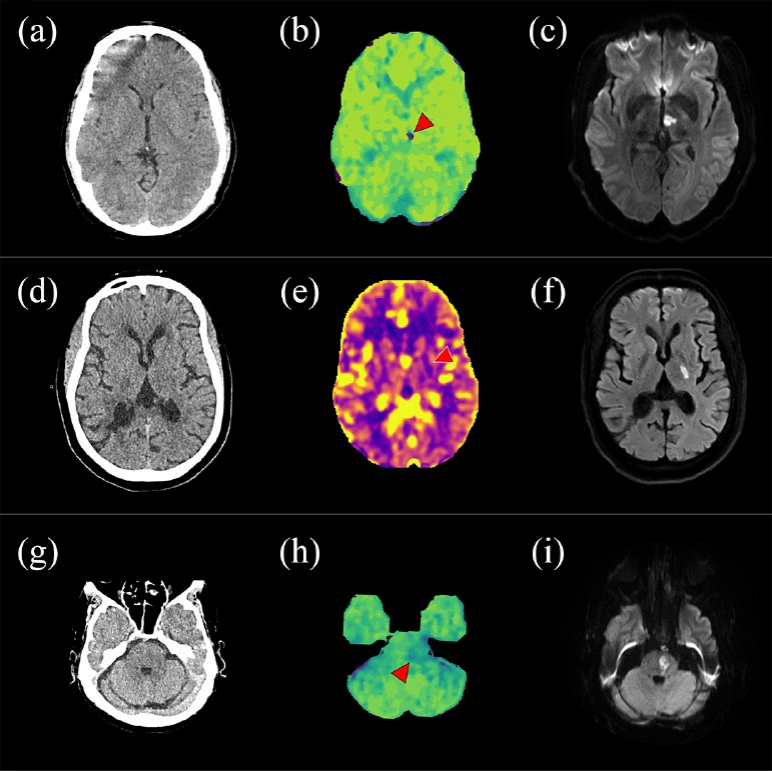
CT perfusion (CTP) in acute lacunar ischemic stroke. A 45-year-old male presented with slurred speech, confusion, and right hemiataxia. Acute ischemia was not identified on the non-contrast CT (NCCT, a) but CTP revealed an area of increased Tmax in the left paramedian thalamus (b, red arrow) that corresponded to diffusion restriction on diffusion-weighted imaging (DWI, c). A 60-year-old male presenting with dysarthria and left-sided facial weakness. An acute infarct was not identified on the NCCT (d), but the CTP cerebral blood flow was lowered in the left internal capsule (e, red arrow), which corresponded to an acute infarct shown on DWI (f). A 70-year-old patient presented with right-sided weakness and dysphagia. NCCT showed a subtle ill-defined region of low attenuation that was indistinguishable from chronic small vessel disease (g). However, CTP showed an area of increased mean transit time in the left hemipons (h, red arrow), which corresponded to an acute infarct on DWI (i).
